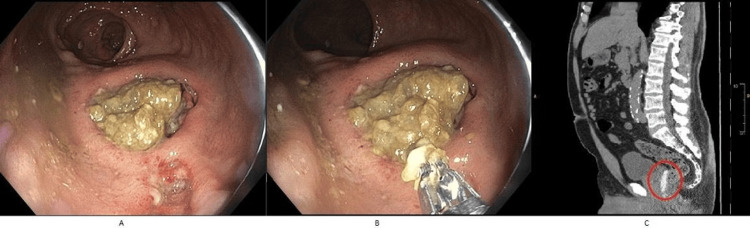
Imaging and endoscopic features of rectal ulceration following SpaceOAR hydrogel insertion (A) Colonoscopy image showing focal proctitis and a large ulcer on the anterior rectal wall. (B) Friable rectal mucosa at the ulcer site, not amenable to biopsy. (C) Axial CT scan showing hydrogel spacer between the prostate and anterior rectal wall with signs of focal proctitis
